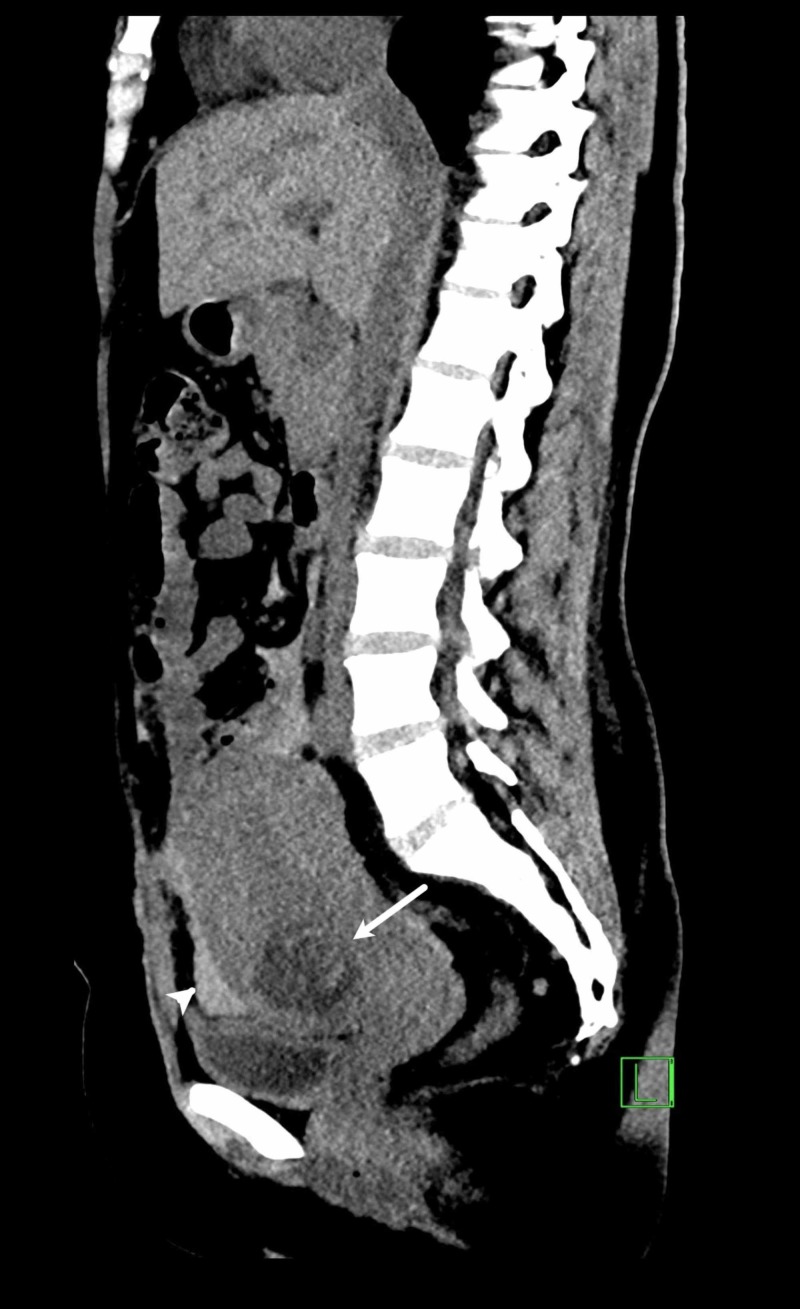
CT Scan for Hemoperitoneum Sagittal CT scan image demonstrating the gestational sac (arrow) and high-density fluid collection (arrow head) suggestive of hemoperitoneum.
